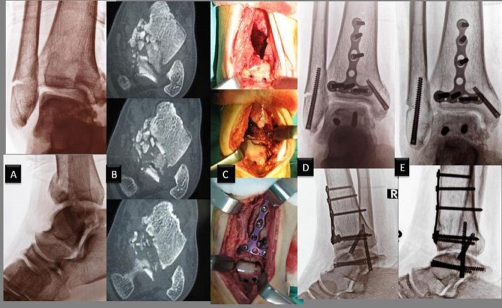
Radiological and intra-operative data's of Case 3. Anteroposterior and lateral radiographs of the ankle of a 33-year-old male who had sustained a comminuted talar body fracture as the result of a fall from a height. Fractures were identified at the right tibia pilon (AO/OTA type C1), lateral malleolar fracture and talus body (OTA class. 81 C3) (A). CT scanning revealed severe comminution of the posterior part of the body (B). Intraoperative photographs demonstrate an anterior approach the fractures are seen before and after the reduction. The fractures were fixed with multiple headless compression screw and locking plate system for distal tibia (C). Postoperative anteroposterior and lateral plain radiographs (D). 12 months later, the fracture had united with a minimal degenerative changes in ankle joint and without evidence of osteonecrosis (E).
